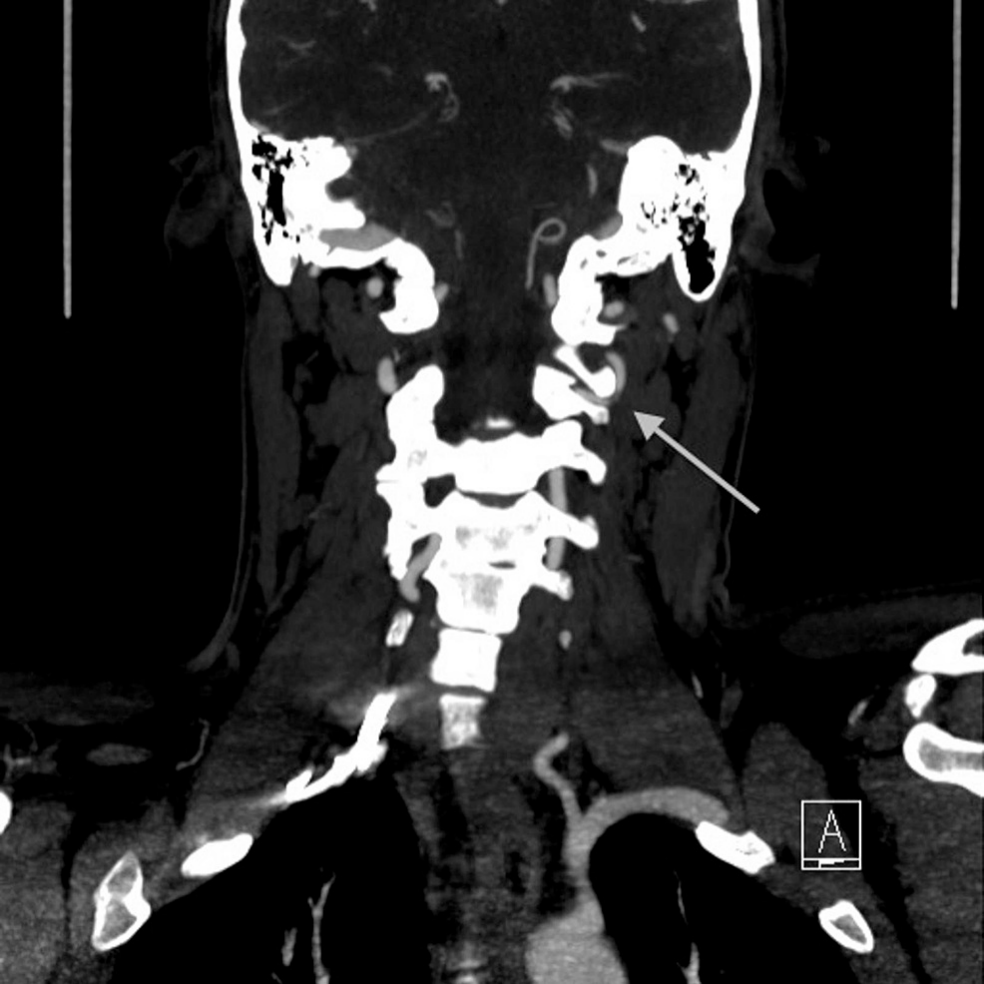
CT angiography (CTA) coronal view, shows severe narrowing of the left vertebral artery at C2 level with possible dissection (arrow).
